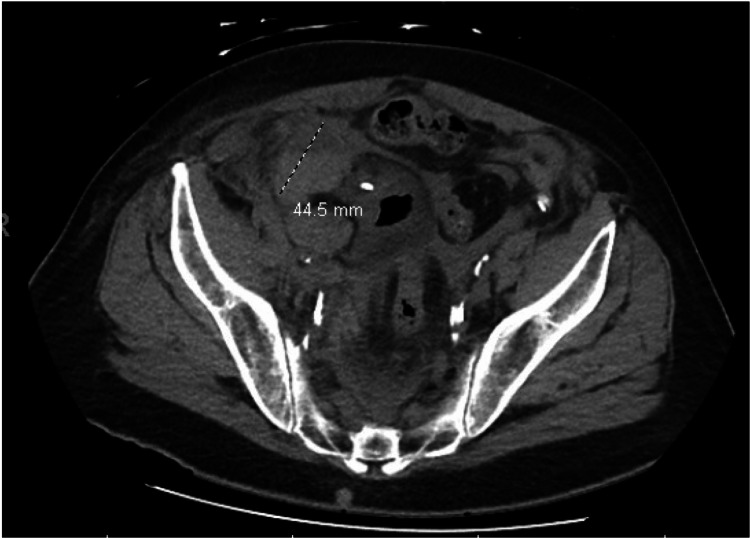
CT abdomen and pelvis demonstrating hemoperitoneum and multilocular hematoma to the right of ureteral implantation
